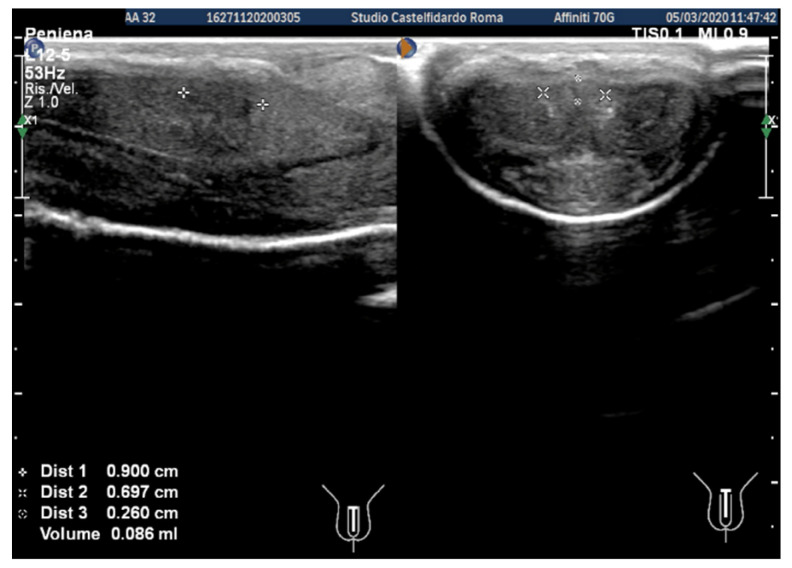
Ultrasonography of the penis after the first treatment cycle (longitudinal and transverse views).
